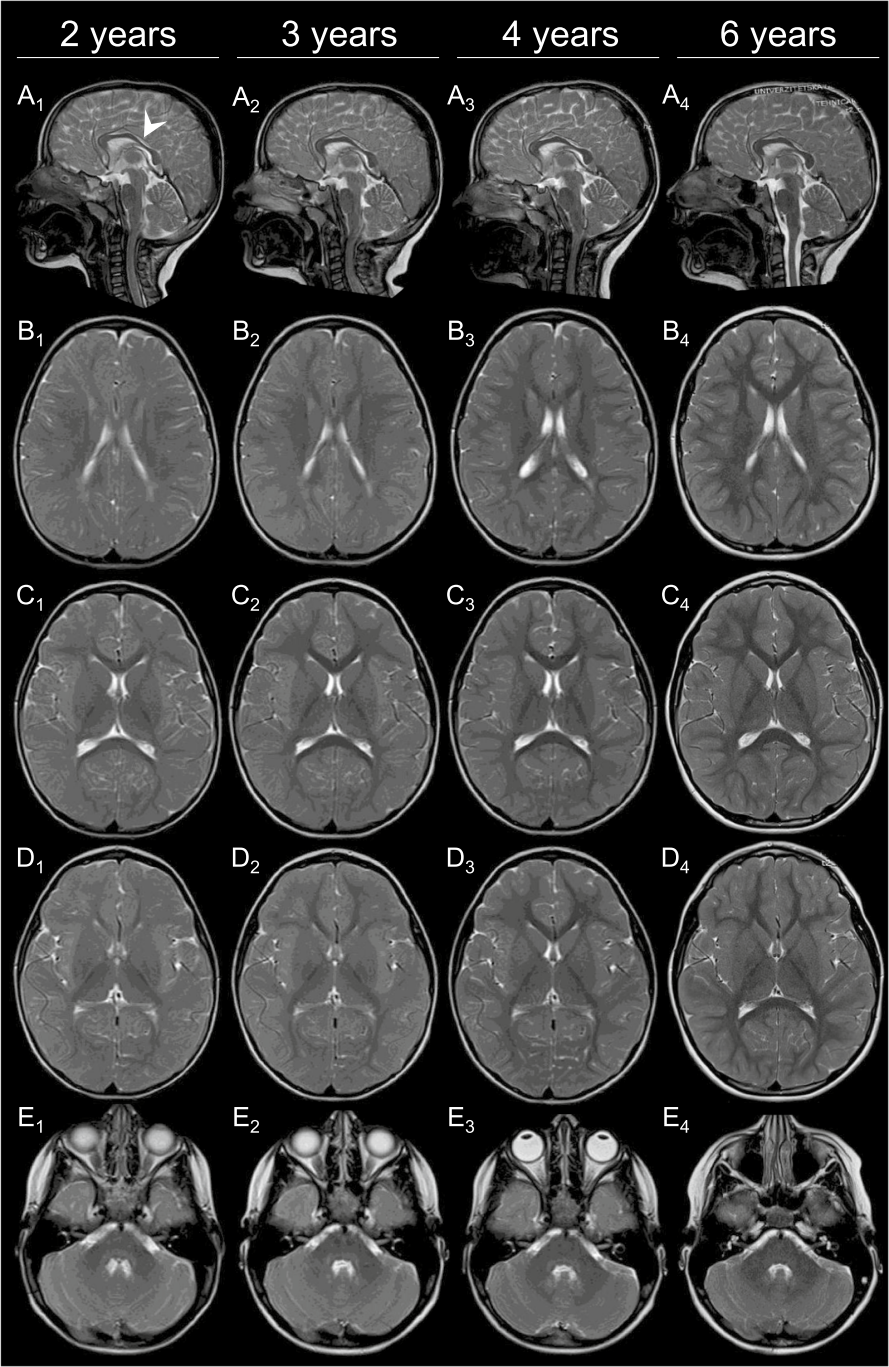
Brain MRI of Patient 2 from age 2 to 6 years. T2-weighted images are shown at 2 years (column 1: A_1_–E_1_), 3 years (column 2: A_2_–E_2_), 4 years (column 3: A_3_–E_3_) and 6 years (column 4: A_4_–E_4_). Sagittal (panel A) images show mild thinning of the corpus callosum (white arrowhead) but otherwise normal midline structures. In panels B, C, D and E, delayed myelination is appreciated, with complete myelination achieved only at age 6 years (B_4_, C_4_, D_4_ and E_4_)
